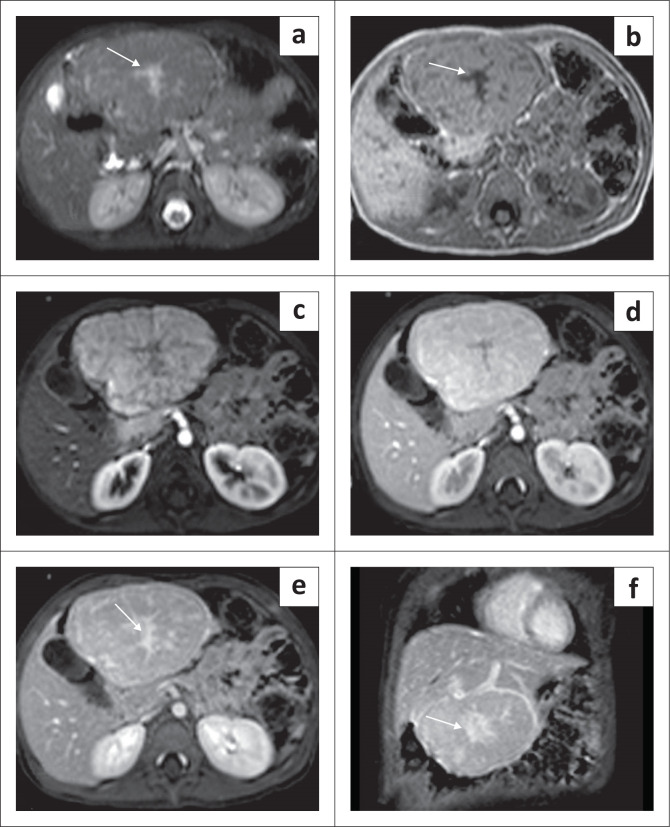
MRI of a 3-year-old female with focal nodular hyperplasia. T2-weighted (T2W) fat saturated axial image of the abdomen showing a well-circumscribed isointense lesion with a hyperintense central scar (arrow in a) in the left lobe of the liver. The lesion is isointense on the T1-weighted (T1W) sequence (b) and the central scar is hypointense (arrow in b). The lesion is hypervascular on the arterial phase (c) and shows contrast retention on portal venous (d) and delayed phases (e). The central scar is hypointense on both the arterial and portal venous phases. Delayed scans axial (e) and coronal (f) image show enhancement of the central scar (arrow in e and f).
